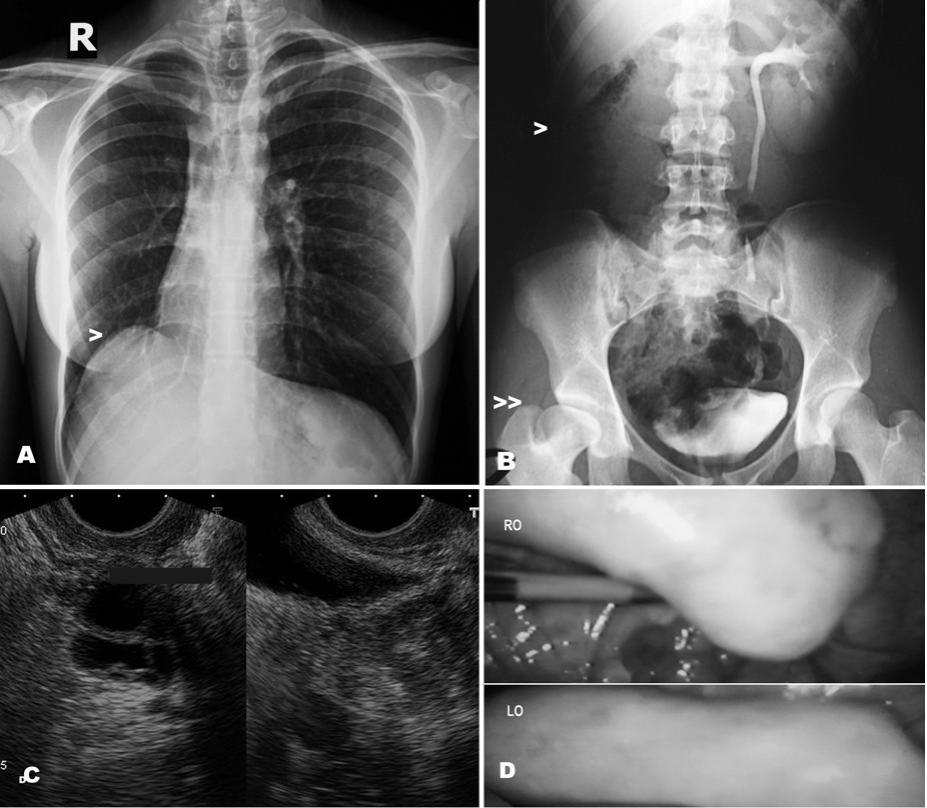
A. Antero-posterior X-ray of the thorax showing right pulmonary hypoplasia. Ascent of the diaphragm (>) and displacement of the heart to the right. B. I.V. pyelography showing right renal agenesis (or dysplasia) (>). The right hip dysplasia is also shown (>>). C. Transrectal ultrasound image of the supposed right ureterocele. D. Laparoscopic images of both ovaries (RO, right ovary; LO, left ovary).
